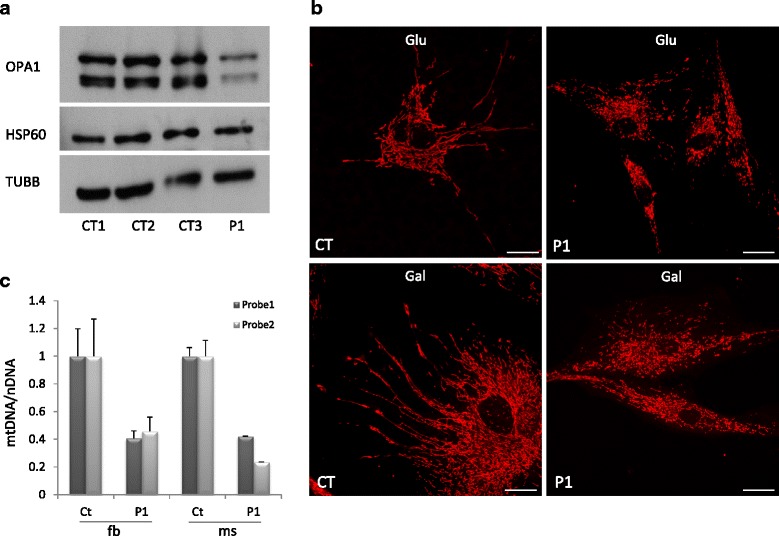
Characterization of fibroblasts from proband 1. a: OPA1 protein amount in patient’s (P1) and control (CT1, CT2 and CT3) fibroblasts, obtained using an anti-OPA1 antibody. Anti-TUBB and anti-HSP60 antibodies were used as loading controls. b: Representative images of mitochondrial morphology (obtained with Mitotracker *red*) in fibroblasts from proband 1 (P1) and a control (CT), grown either in glucose or galactose medium. Scale bar: 20 μm. c Quantification of mtDNA amount in fibroblasts (fb) and skeletal muscle (ms) from proband 1 (P1) and controls. (Ct). The bars represent the amount of mtDNA normalized to nuclear DNA (nDNA), compared to the mean value of controls (=1). Two different probes for mtDNA were used. Data are represented as mean + SD
